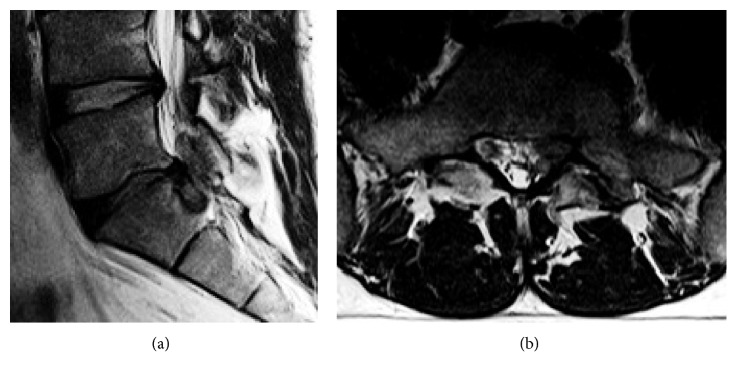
Lumbar MRI of case 1 showing highly migrated disc L5-S1 herniation inside the sacral canal.
